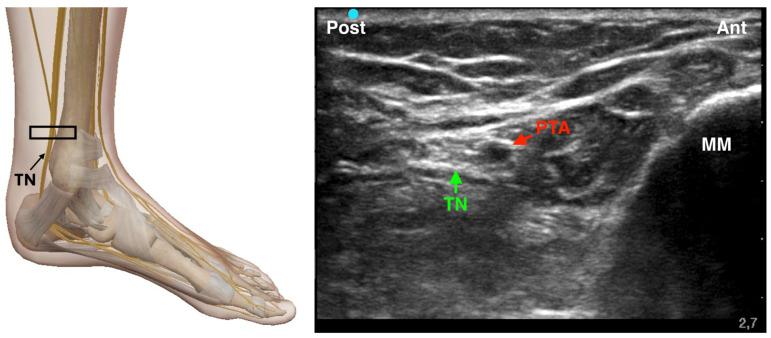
Ultrasound-guided Tibial Nerve Block. TN: Tibial Nerve; PTA: Posterior Tibial Artery; MM: Medial Malleolus; The black rectangle indicates the placement of the ultrasound probe on the anatomical area.
